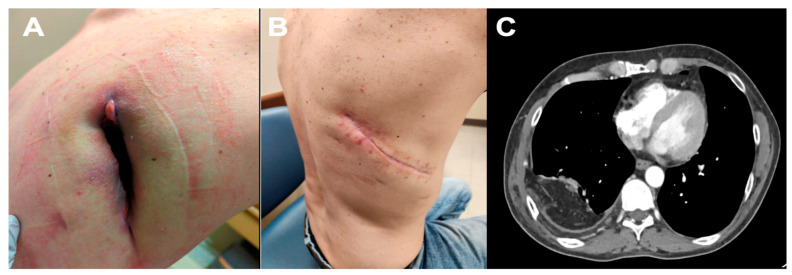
Patient A. (A)—15 × 4 cm chest wall defect after previous thoracostomy for the entrapped lung with bronchopleural fistula. (B)—Complete closure of chest wall defect. (C)—CT thorax with IV contrast demonstrating location of omental flap with coverage of chest wall defect.
